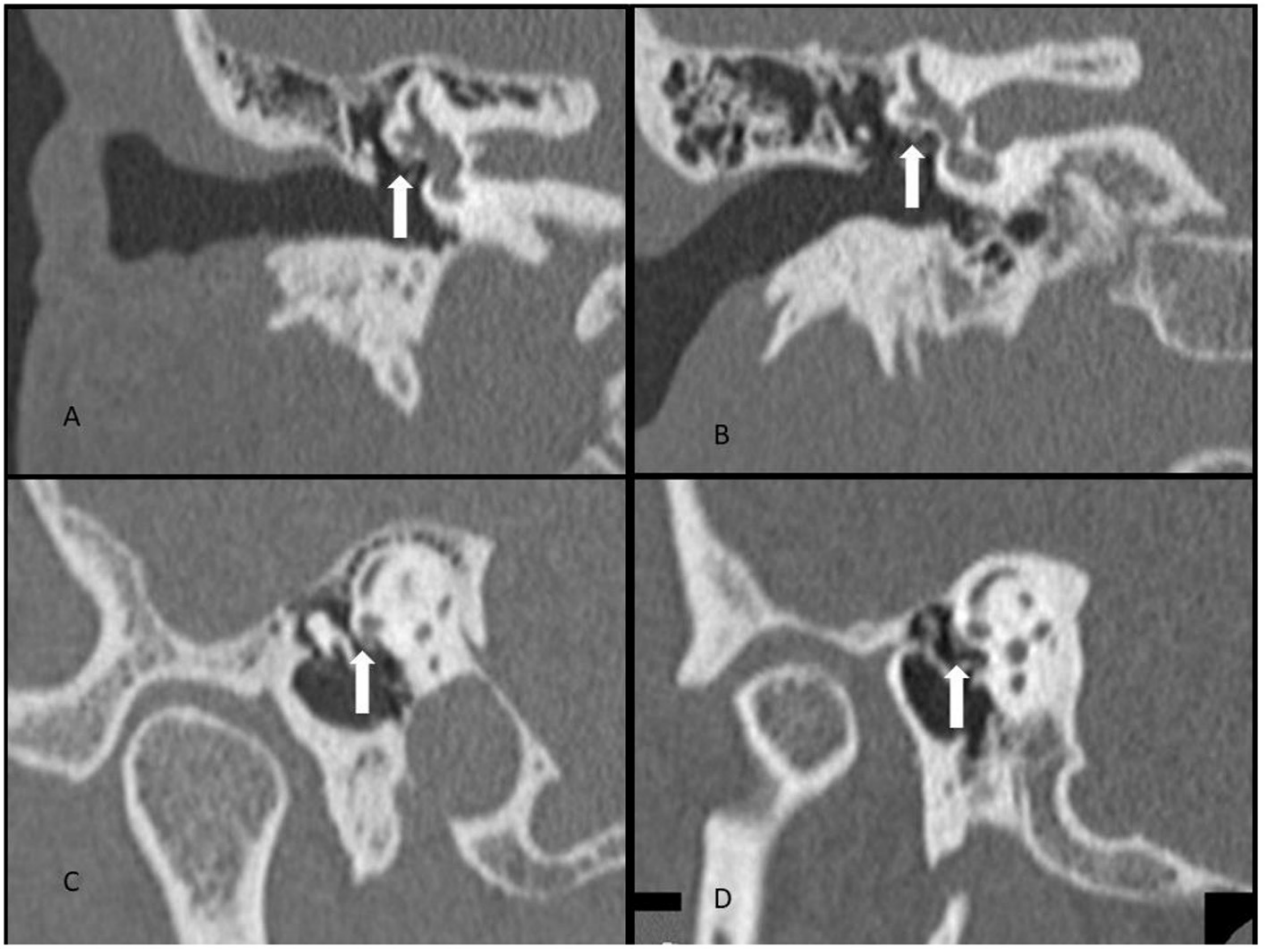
CT scan demonstrating (A) HSC-FND on coronal imaging, (B) normal HSC and facial nerve anatomy on coronal imaging, (C) HSC-FND on Poschl imaging, and (D) normal HSC and facial canal on Poschl imaging.
